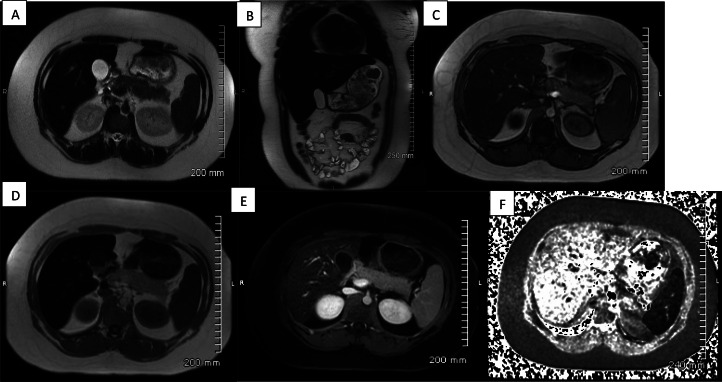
Images from a contrast-enhanced abdominal MRI from 8 years prior. T2 axial (A) and coronal (B) images show diffuse low signal throughout the liver (lower than muscle) consistent with hemochromatosis. T1 opposed-phase (C) and T1 in-phase (D) shows significant loss of signal throughout the liver with the longer echo time (in-phase images). Contrast-enhanced axial T1 image (E) again shows abnormal signal loss throughout the hepatic parenchyma relative to other abdominal organs. Axial R2* map from an iron quantification sequence (F) confirmed severe iron overload.
